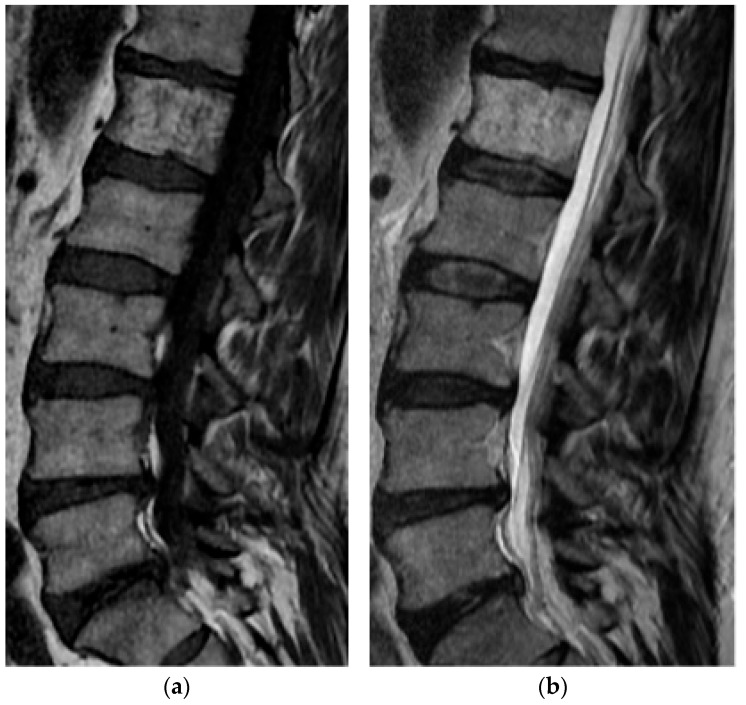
Hemangioma. (a) Sagittal MR T1- and (b) sagittal MR T2-weighted images: the L1 vertebral body hemangioma has increased signal on T1 and T2 secondary to intralesional fat and fluid, respectively. Vertical striations are in keeping with thinned but intact trabeculae.
